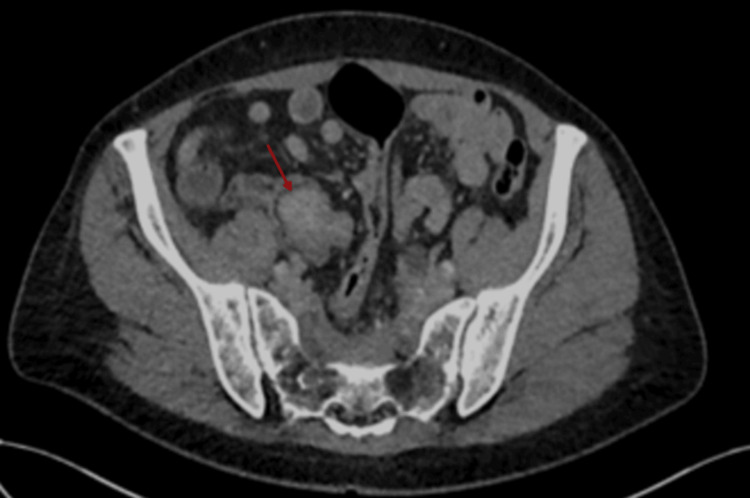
Initial CT of the thorax/abdomen/pelvis on 28 August 2024 showing retroperitoneal fibrosis and an enhancing lesion at the ileocecal junction (red arrow).
